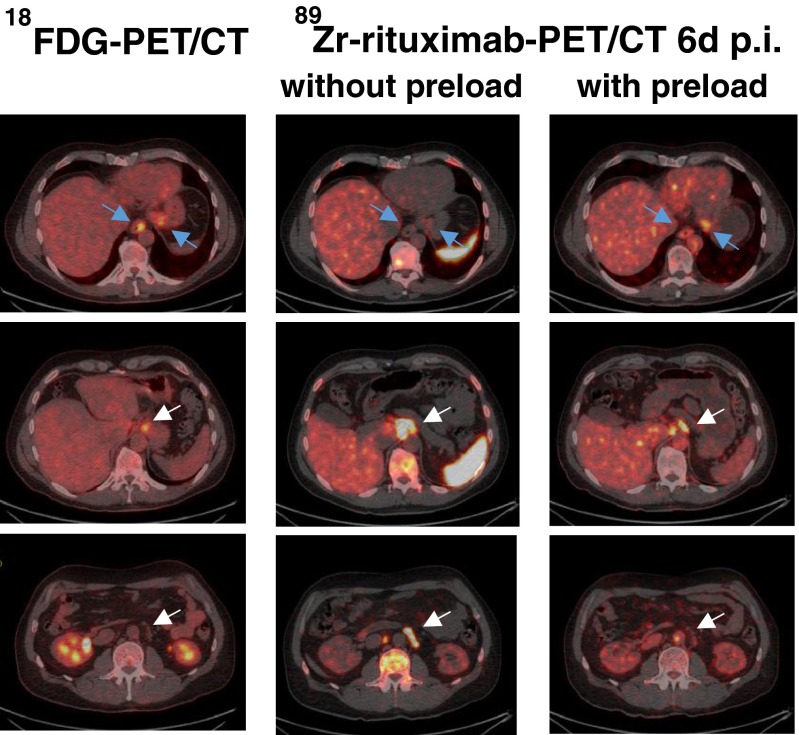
^18^F-FDG PET/CT and ^89^Zr-rituximab immuno-PET/CT images in patient 2 obtained 6 days after injection with and without a preload of unlabelled rituximab show lower tracer uptake in involved lymph nodes with the preload (*white arrows*), but higher uptake in less accessible visceral lesions (oesophagus and stomach; *blue arrows*) resulting in better tumour targeting
